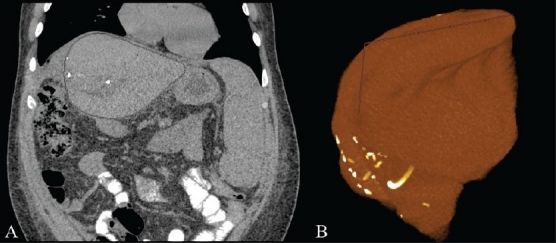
Coronal CT scan (A) and segmented volume rendered (B) images of a partially resected liver. With this technique, it is possible to determine the volume of the residual liver in milliliters, essential clinical information for the post-operative patient
